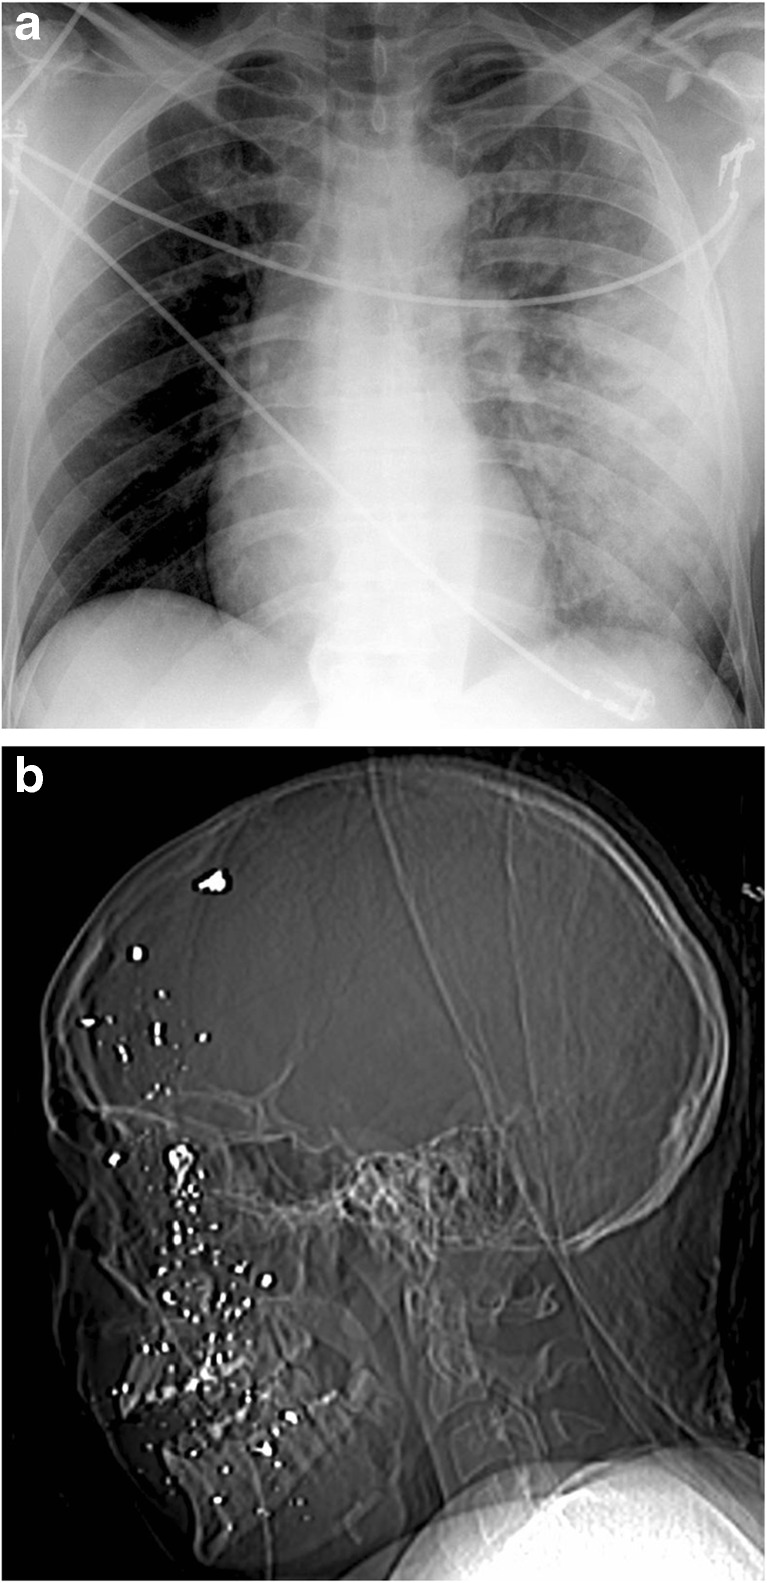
Imaging of two patients in the emergency department. a Plain film of the chest of a patient with blast injury to the upper left chest causing pulmonary contusion, comminuted fracture in the lateral part of the clavicle and multiple rib fractures. b CT scout of patient from the shooting incident with fragmenting injury to the face and head
